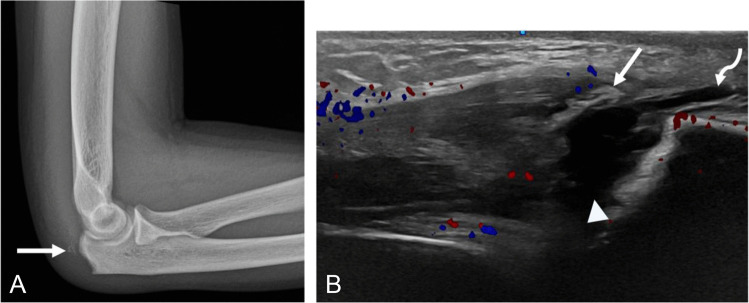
A 15-year-old girl with acute elbow pain after hearing a “pop” while throwing a football. A Lateral radiograph of the elbow shows an avulsed ossific fragment (*arrow*) dorsal to the olecranon. B Ultrasound demonstrates a complete retracted tear of the triceps tendon with a fluid- and blood-filled gap (*arrowhead*) and the avulsed ossific fragment at the olecranon insertion (*arrow*). Note the associated fluid distention of the olecranon bursa (*curved arrow*)
